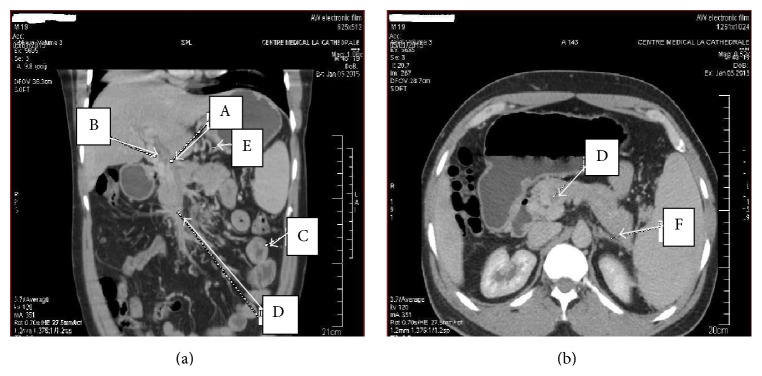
Abdominal contrast-enhanced CT scanner: control after three months on oral anticoagulation. (a) Partial recanalization of the portal venous thrombosis (A) and the superior mesenteric venous thrombosis (D), the portal cavernoma (B) and gastric collateral vessels (E), and thickening of the small intestinal bowel (C). (b) Superior mesenteric venous partial recanalization (D) and residual inflammatory flows (F). Light Speed CT-16 (GE Medical Systems, USA), contrast: iobitridol 300 mg/mL (Xenetix, Guerbet, Roissy CDG Cedex, France). With the permission of the Cathedral Medical Center (CMC), Yaoundé.
